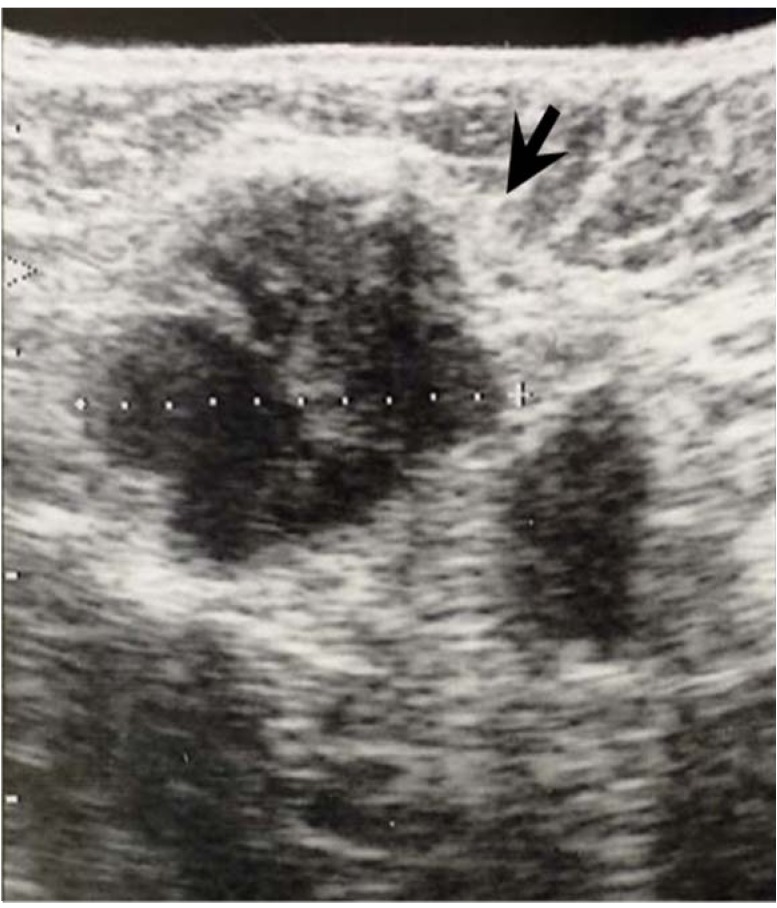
Ultrasonographic appearance of type 3 lymph node metastases. This lymph node (arrow) shows clearly defined boundaries but scattered large internal echoes accompanied by notching.
